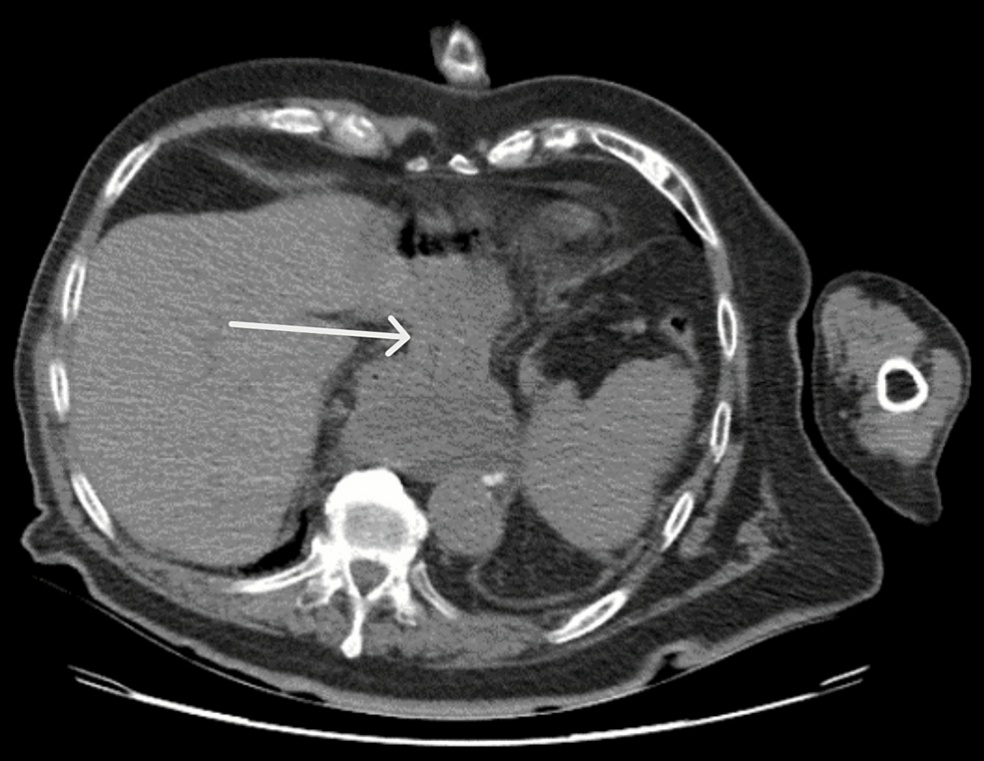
CT scan of the abdomen in the axial plane, showing twisting of the gastric pouch at the level of the diaphragmatic opening
